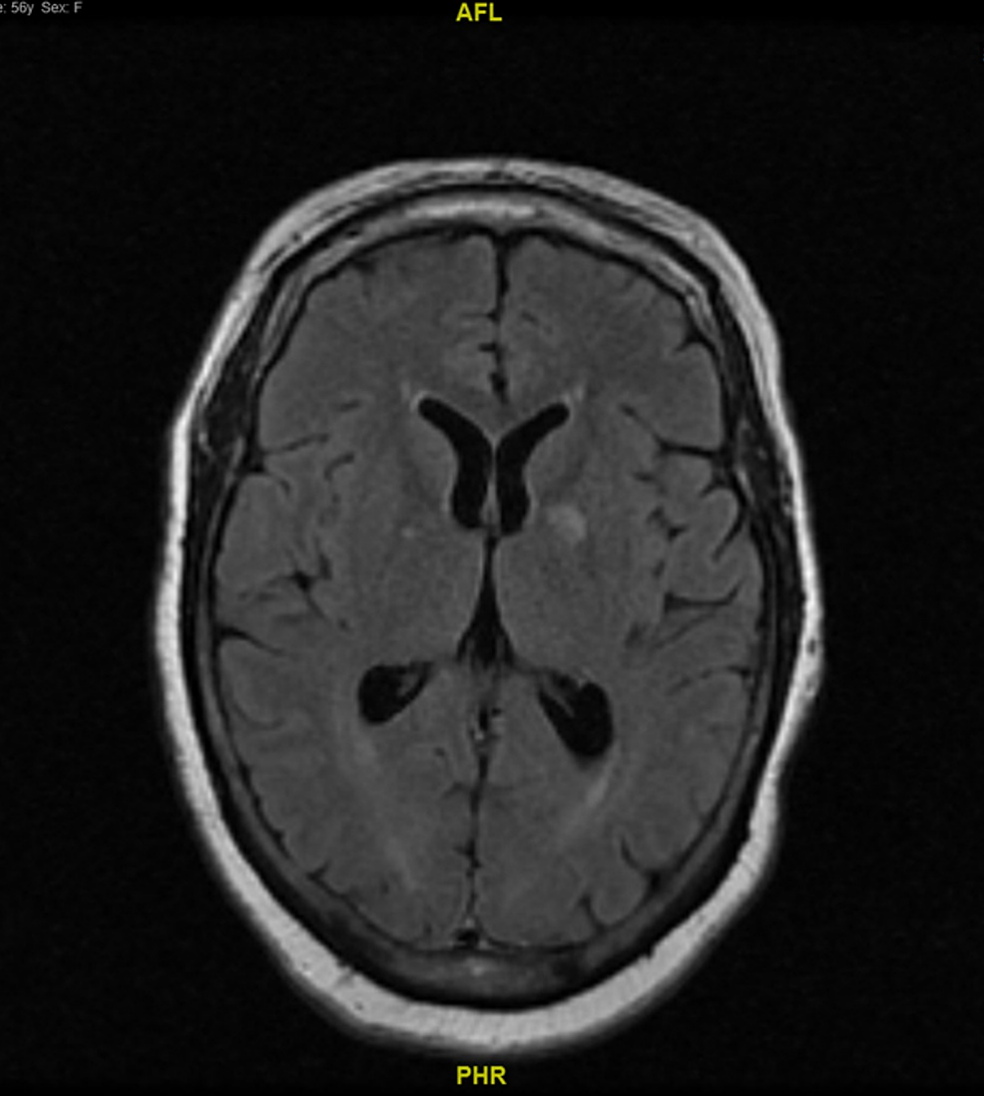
MRI of the brain without contrast in axial view on the day of admission The image shows no obstruction in the ventricles.
